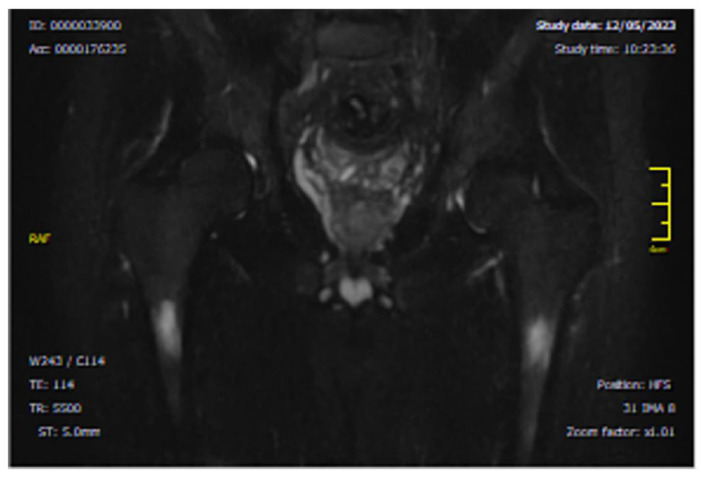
Coronal MRI section, showing bilateral intra-spongious BME of the femoral diaphysis. The image shows a focal bilateral hyperintense area on STIR sequence (T2-weighted with fat suppression) in the femoral diaphysis.
